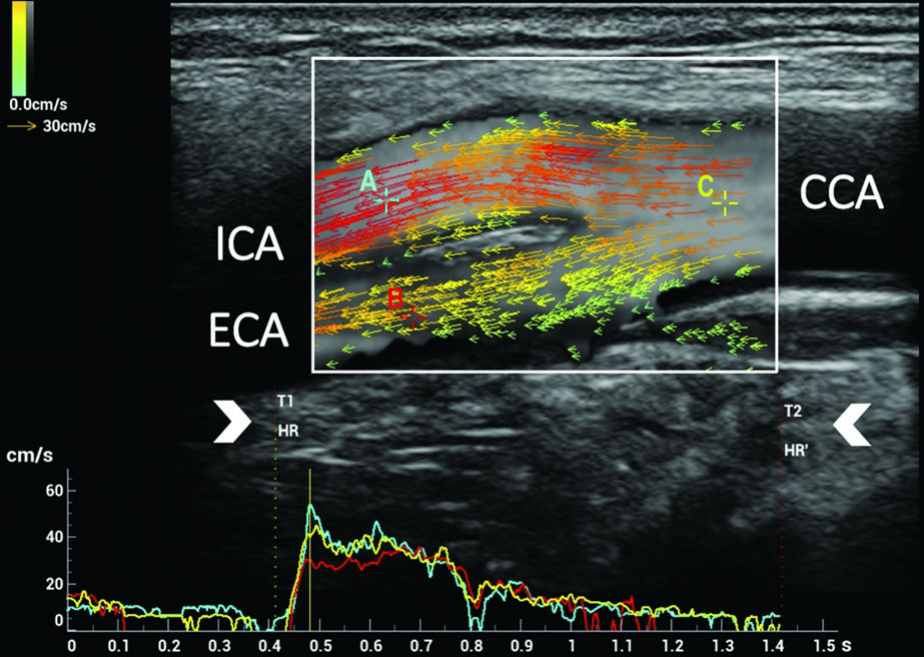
Ultrasound vector flow imaging of laminar flow in the CB. In the upper part, a single image, corresponding to the systolic peak, was extracted from the 900 images of the cine loop acquired in 1.5 seconds. The system measures the speed and direction of all blood cells flowing through every point of the selected region of interest. The flow is represented by several color arrows. The color and size of the arrows allow visual quantification of flow velocity in the 2D space. The arrows are randomly distributed and flow according to their velocities. At a static image (a single frame), different concentrations of the arrows in the lumen could be seen. In the lower part, the flow velocity‐versus‐time curves were measured in 3 locations of the CB: internal carotid artery (ICA) curve (A) displayed in light blue, external carotid artery (ECA) curve (B) in red, and common carotid artery (CCA) curve (C) in yellow: the time interval (T1–T2) corresponding to the cardiac cycle duration (arrowheads) can be selected manually on the acquired row data video clip, thus allowing a correct subsequent WSS measurement. In the updated version, the HR and HR′ (as shown in the image) allow the selection of the corresponding cardiac cycle duration automatically or manually.
